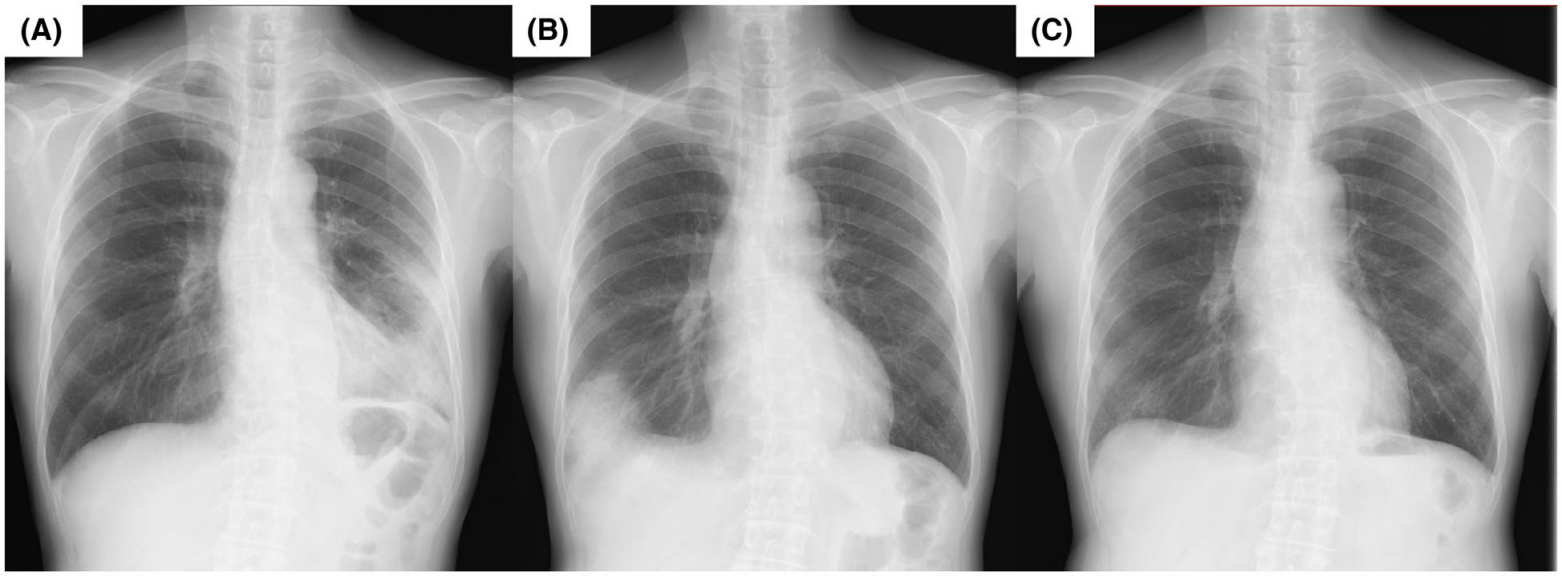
Chest radiographs before vaccination (A: September 2020), 2 days after COVID‐19 mRNA vaccination (B: July 2021) and after treatment (C: September 2021). After vaccination, infiltrative shadows in the right lower lobe were visible. These findings ameliorated after the treatment
